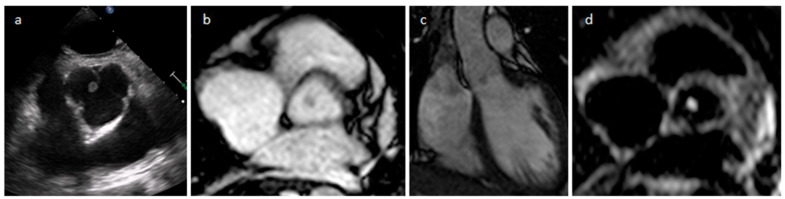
A 28-year-old male patient with an aortic cusp fibroelastoma during transesophageal echocardiography (a), iso/hypointense on axial (b) and left ventricular outflow tract (c) cine sequences, and hyperintense on axial T2-weighted images (d).
